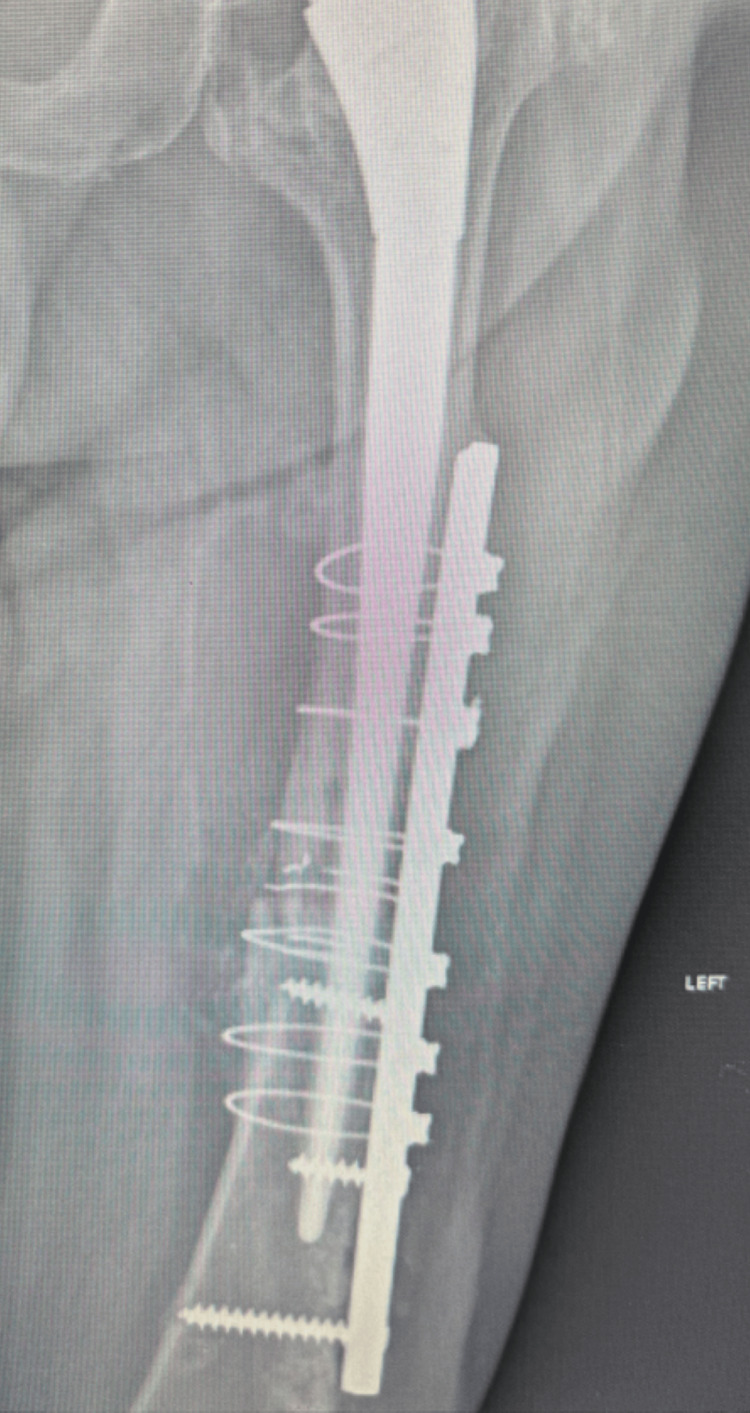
Postoperative radiograph demonstrating the final result following insertion of a long femoral stem for hemiarthroplasty and ORIF of the femoral osteotomy site using a lateral plate, screws, and cerclage wires ORIF: open reduction with internal fixation
